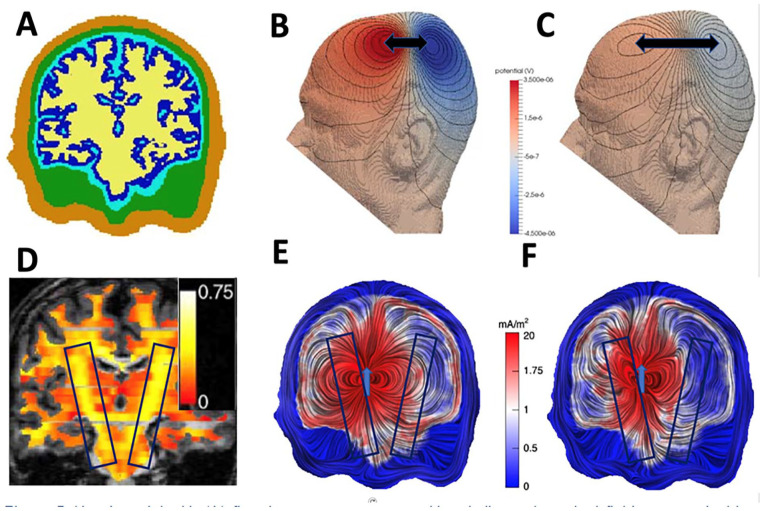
Head model with (A) five tissue compartments skin, skull, cerebrospinal fluid, gray matter, and white matter. (B, C) Isopotential lines for a mainly tangentially oriented source in somatosensory cortex where the underlying model in (B) has 10 times higher skull conductivity than in (C). The resulting smaller distance between potential peak and trough in (B) as compared with (C) are indicated by black arrows. (D) fractional anisotropy based on diffusion tensor magnetic resonance imaging (MRI) for the white matter compartment. Volume currents for a thalamic dipole source in the head model with (E) isotropic and (F) 1:10 anisotropic white matter conductivity anisotropy (reprinted from Wolters and others 2006) Copyright, with permission of Elsevier).
